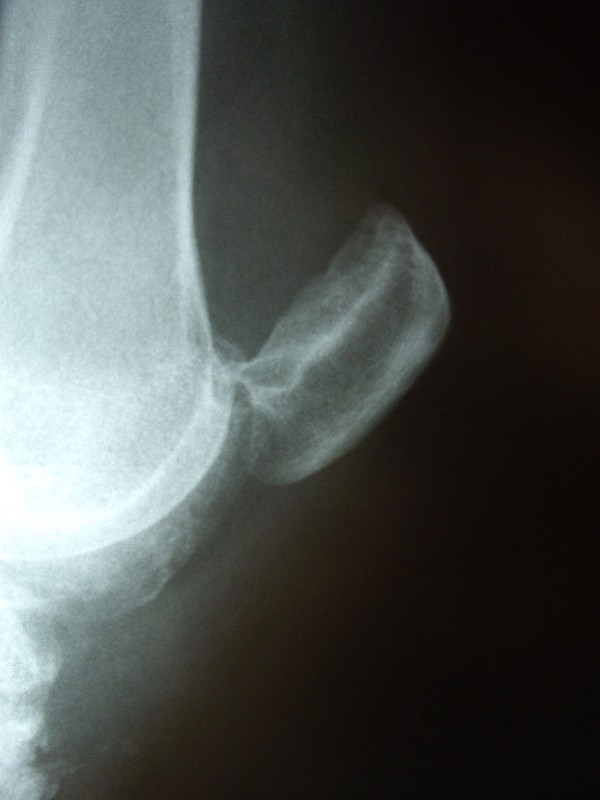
Lateral radiograph showing the kneecap in superior dislocation with blocking in the osteophytosis of the inferior patellar pole and femoral osteophyte.
